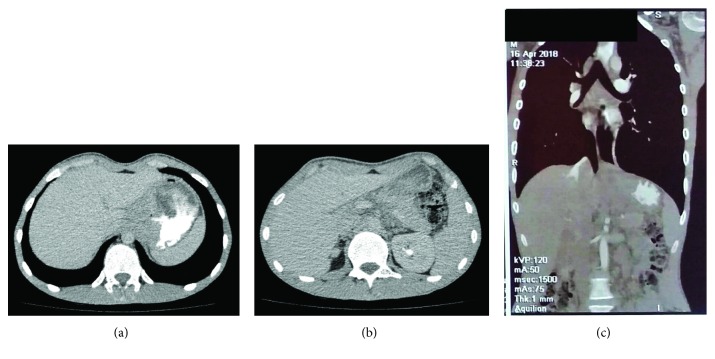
CT scan series with a radiocontrast agent showing a mass in the level of the stomach fundus invading the surrounding fatty tissue between the liver and the stomach without any liver metastasis. (a), (b) Axial sections of the abdomen. (c) Coronal section of the chest and the abdomen.
